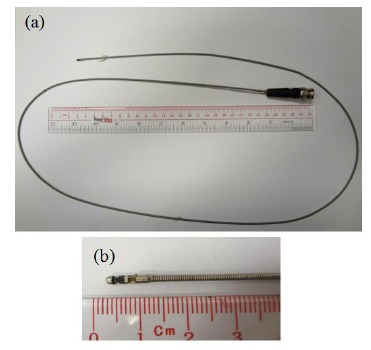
The prototype of a 10 MHz rotatable ultrasonic (a) catheter and (b) enlarged probe [64].
